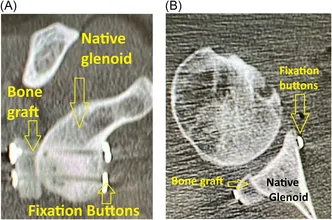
(A,B) Immediate postoperative computed tomography (CT) scans showing correct positioning of the posterior iliac crest bone graft flush with the glenoid surface and with a large bone‐to‐bone contact area. Stable fixation with cortical buttons and the use of an autograft optimize conditions for graft incorporation and long‐term restoration of posterior glenoid bone stock.
